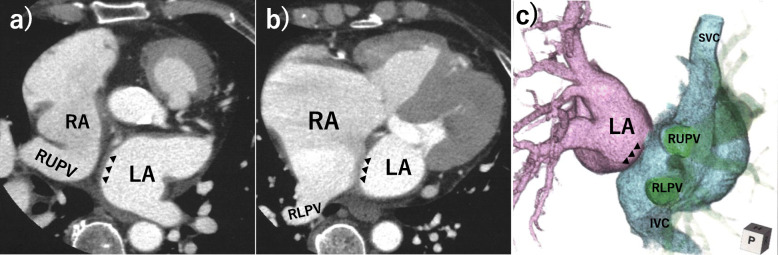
Preoperative multidetector computed tomography angiography. Preoperative multidetector computed tomography angiography demonstrating the anomalous drainage of both the right upper and lower pulmonary veins into the right atrium. The intact atrial septum (black arrowheads) is small and the plane is displaced leftward. Axial images (a, b) and three-dimensional reconstruction (c) are presented. LA = left atrium, RA = right atrium, RUPV = right upper pulmonary. Vein, RLPV = right lower pulmonary vein, SVC = superior vena cava, IVC = inferior vena cava
